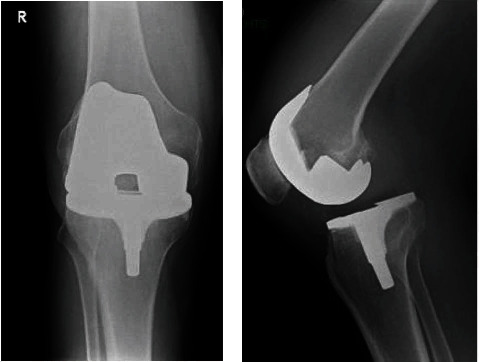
(a) Anteroposterior radiograph of case 2 at presentation in the emergency room. (b) Lateral radiograph of case 2 at presentation in the emergency room.
